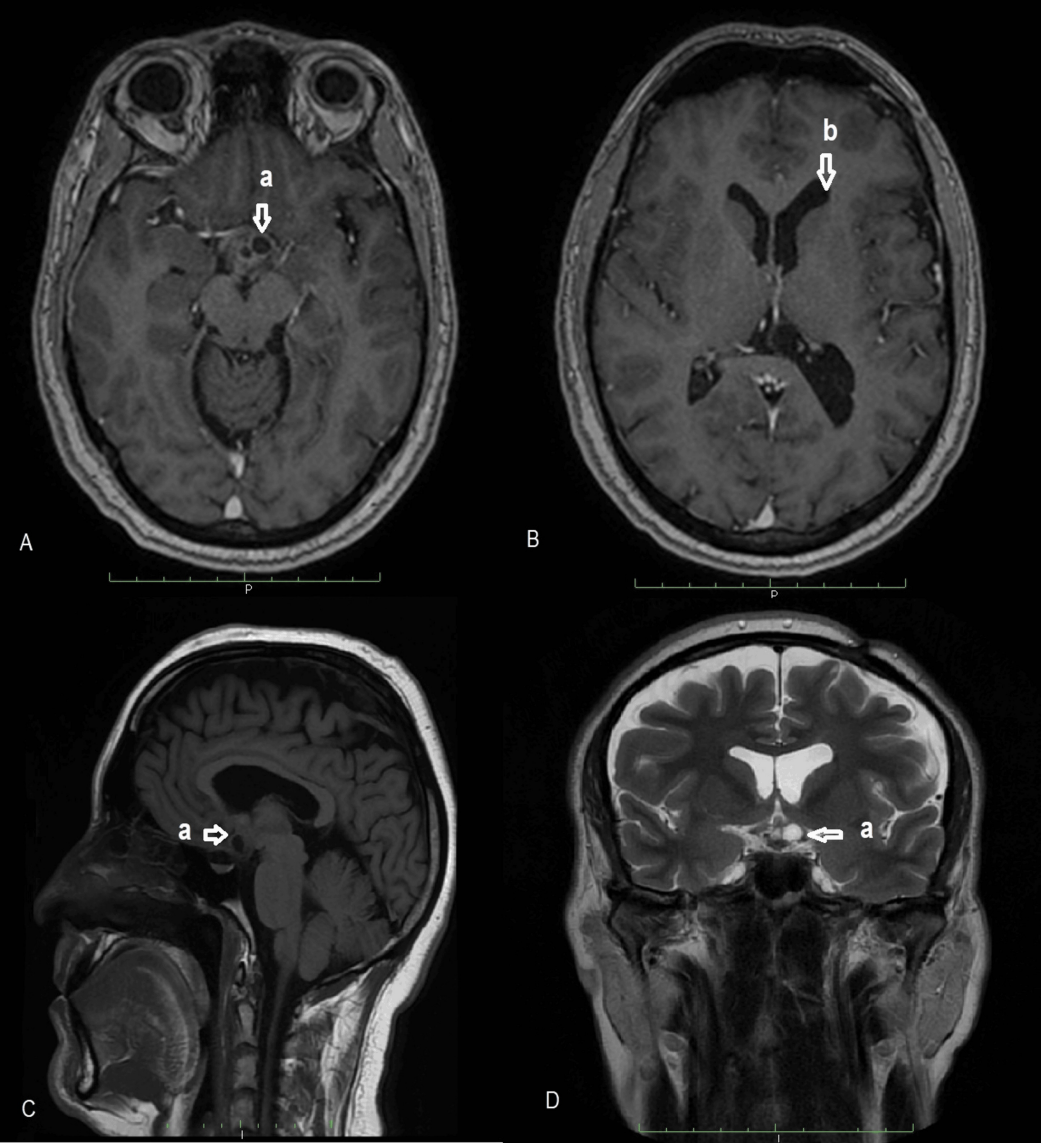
Follow-up brain MRI of case 1 showing residual tumor at the floor of the third ventricle with regression of hydrocephalus. A: Postoperative axial T1-weighted brain MRI sequences showing a residual tumor located at the floor of the third ventricle. B: Postoperative axial T1-weighted brain MRI sequences showing a regression of hydrocephalus. C: Postoperative sagittal T1-weighted magnetic resonance imaging (MRI) sequences reveal a residual tumor along the surgical site. D: Postoperative coronal T2-weighted MRI sequences showing a residual tumor infiltrating the floor of the third ventricle. a: residual tumor. b: regression of hydrocephalus
